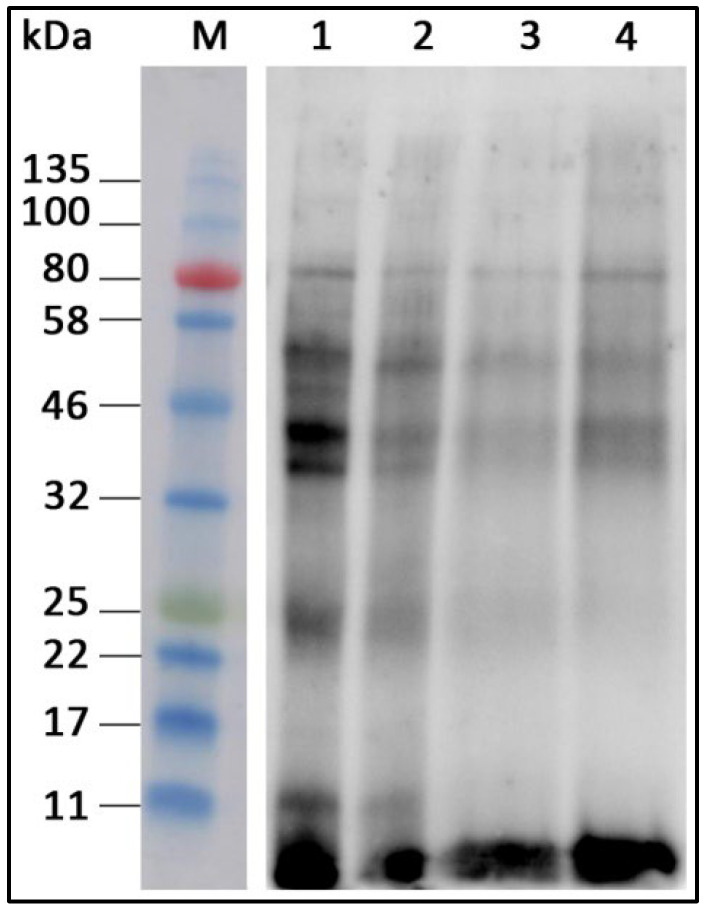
Western blot detection of fennel proteins. M: molecular mass standard; 1: untreated protein extract; 2: protein extract treated with an ultrasonic bath; 3: protein extract treated with an ultrasound probe; 4: protein extract exposed to a temperature of 95 °C.
